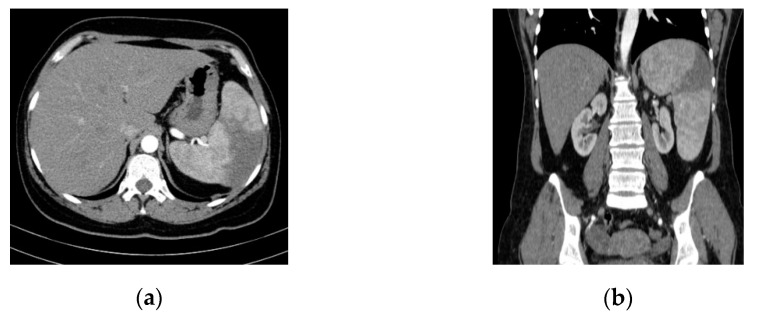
Abdominal/pelvic contrast-enhanced CT scan (Optiray™ 350). Splenomegaly and splenic infarction are depicted in (a) axial and (b) coronal sections.
